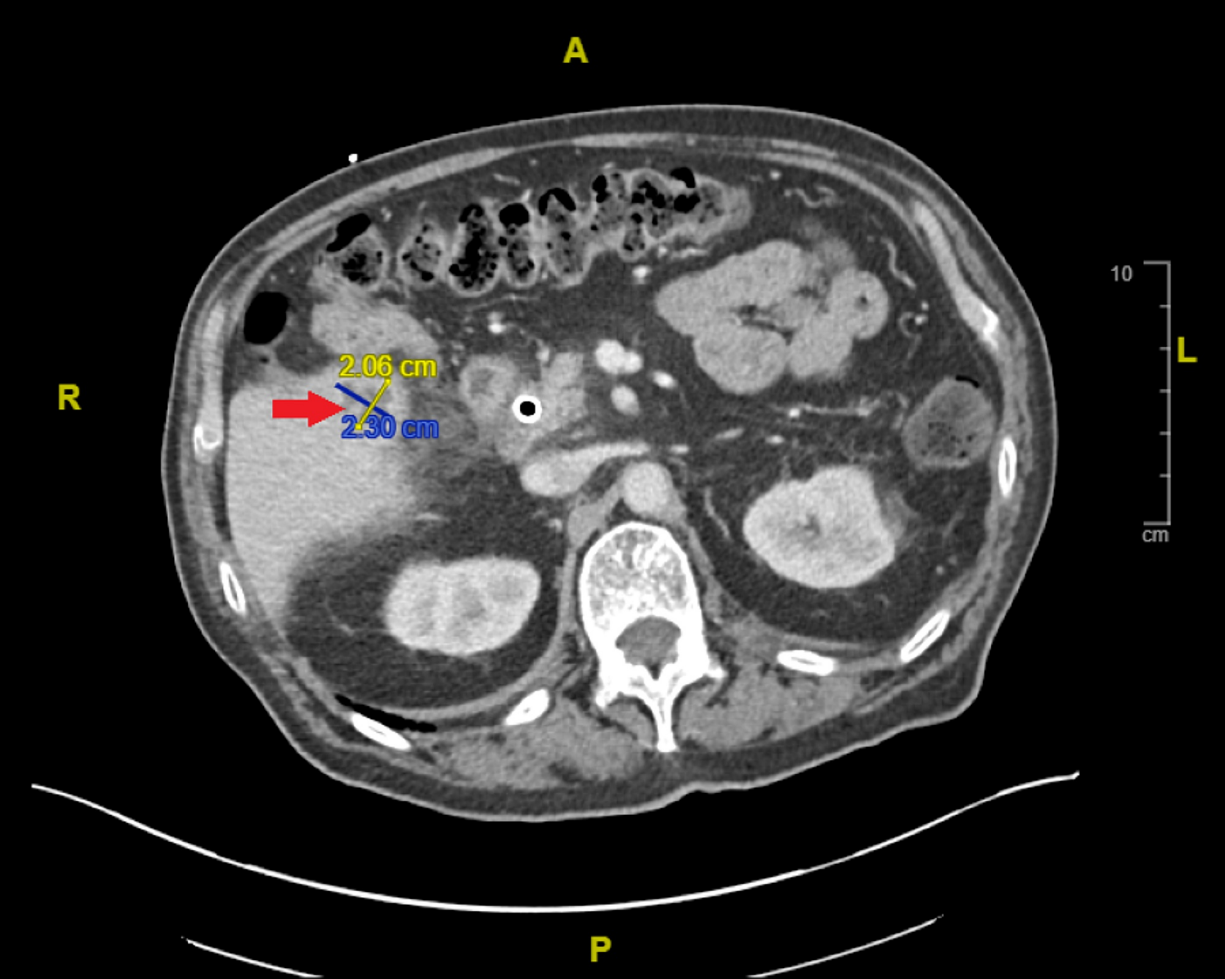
Cross-sectional computed tomography (CT) of abdomen and pelvis with intravenous contrast Red arrow: Findings suggestive of cholangitis with persistent mild right intrahepatic biliary dilation presumably due to stricturing upstream to the common duct stent and new multilocular rim-enhancing segment V lesions concerning for evolving biliary abscesses measuring up to 2.3 cm
